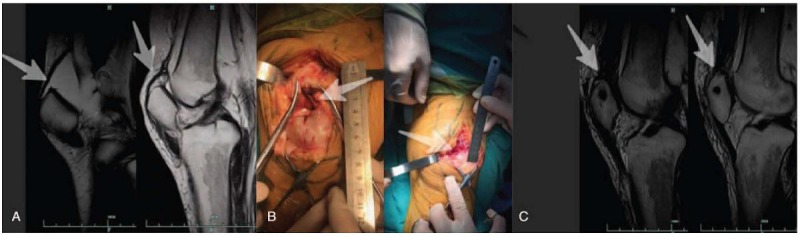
(A) Preoperative T2-weight sagittal MRIs of both knees clearly show the rupture at the osteotendinous junction as indicated by the arrows. (B) Intraoperatively, bilateral complete rupture between quadriceps tendon and patella was identified. (C) Postoperative sagittal T2-weighted MRIs of the knees revealed the normal continuity of the repaired quadriceps tendon with the superior pole of patella. MRIs = magnetic resonance images.
